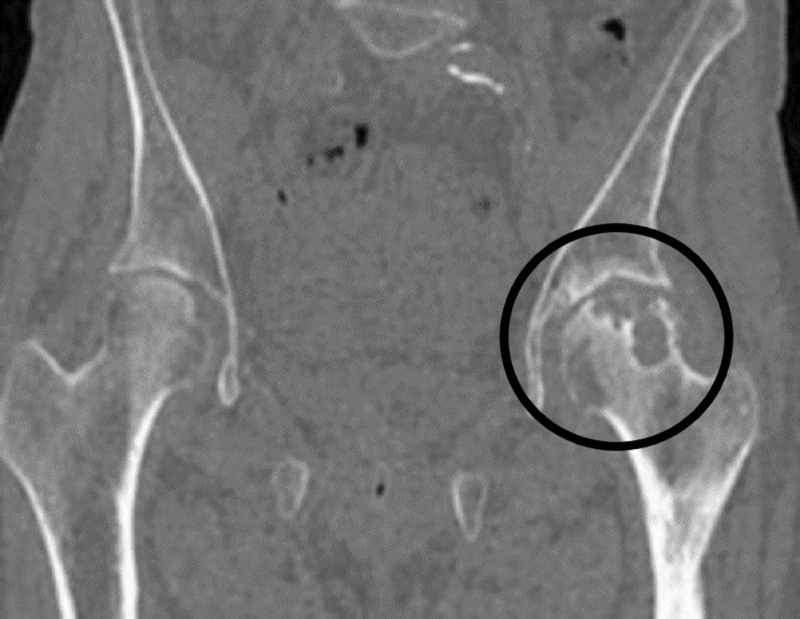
CT pelvis - coronal view Osteonecrosis left hip encircled.
